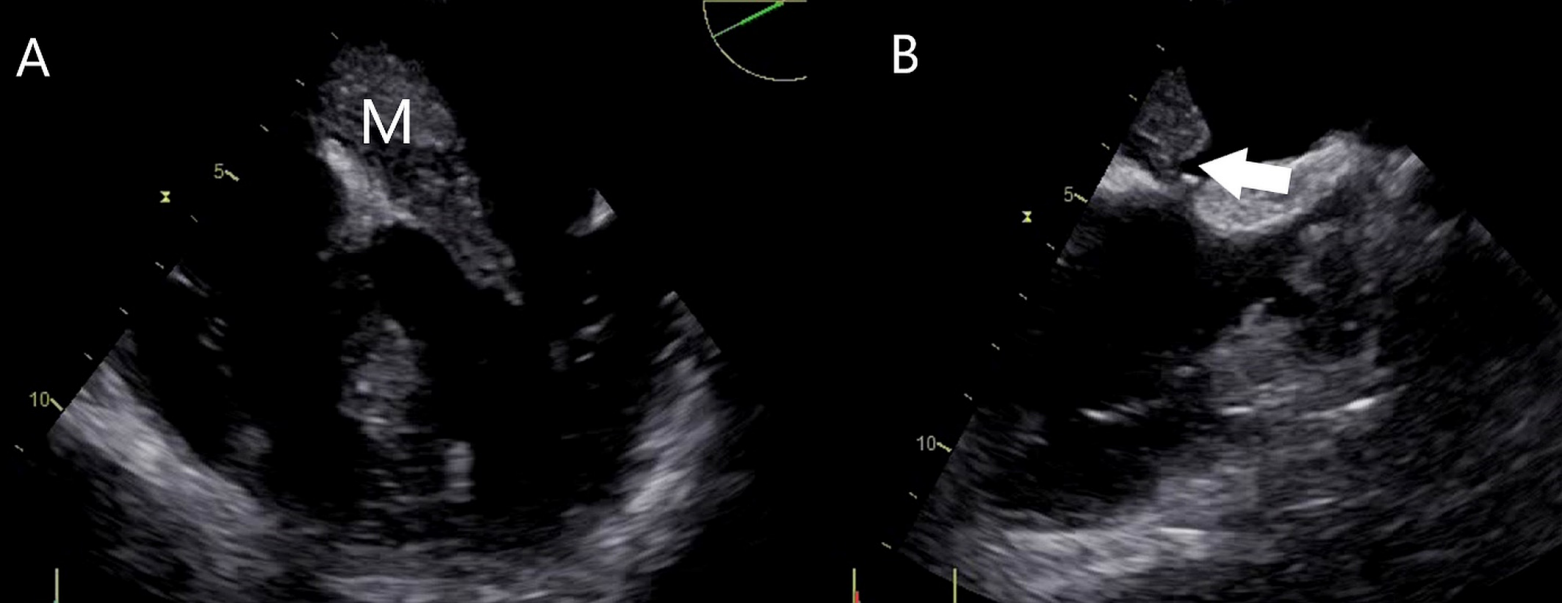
Transesophageal echocardiography shows a large left
